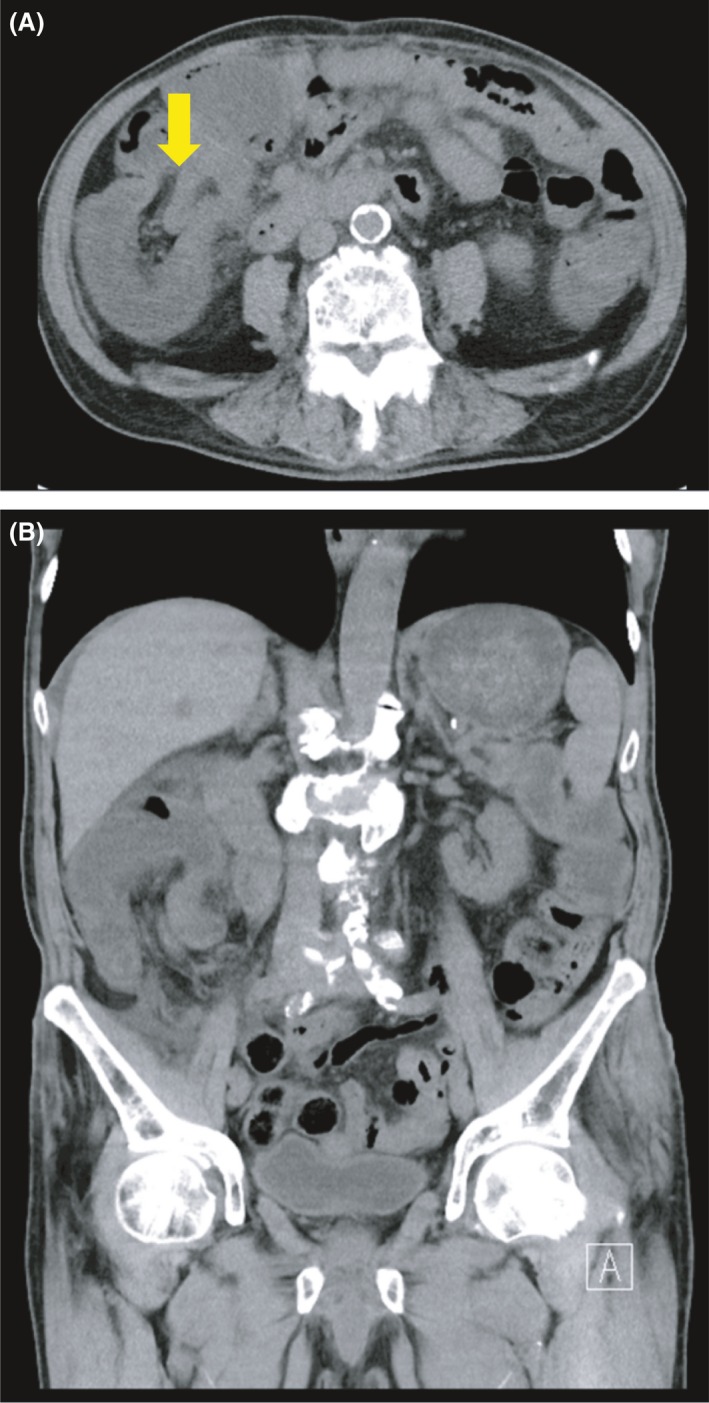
(A, B) CT finding. A plain CT scan of the abdomen and pelvis revealed a pseudokidney sign from the terminal ileum to the ascending colon.
